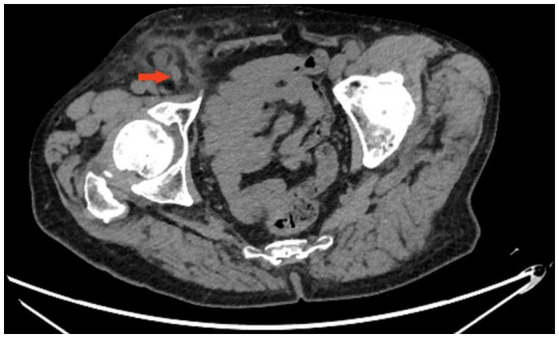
Abdominal CT axial scans reveal an appendix within a right inguinal (Amyand’s) hernia.
